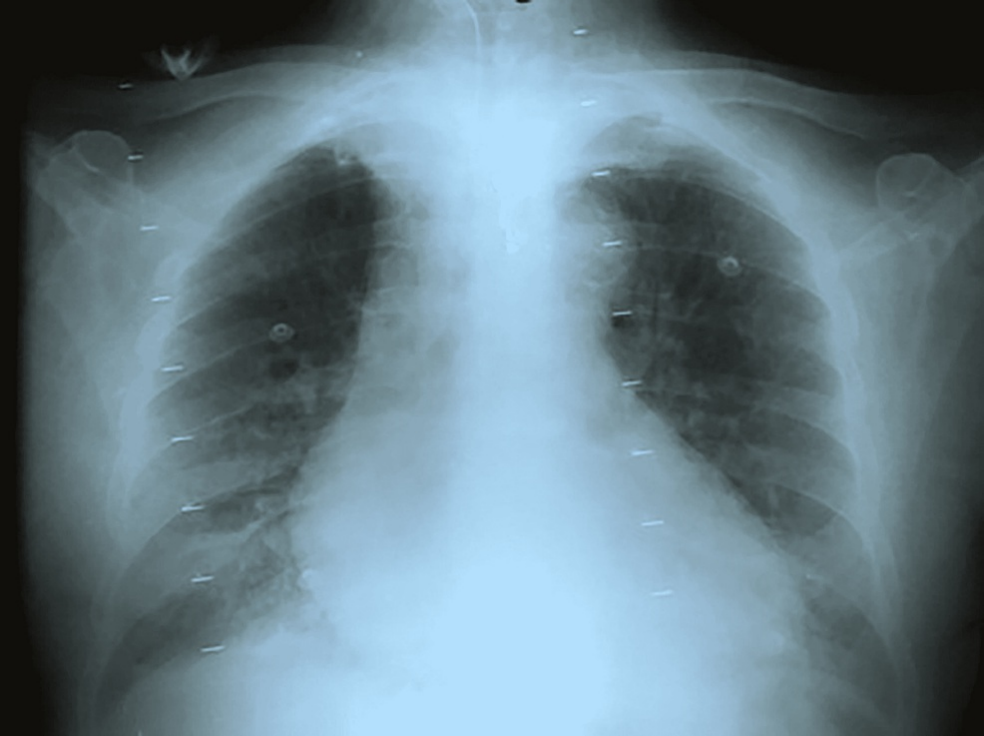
Chest plain radiography (portable) showing bilateral diffuse pulmonary opacities and cardiomegaly suggestive of ARDS. ARDS: acute respiratory distress syndrome
